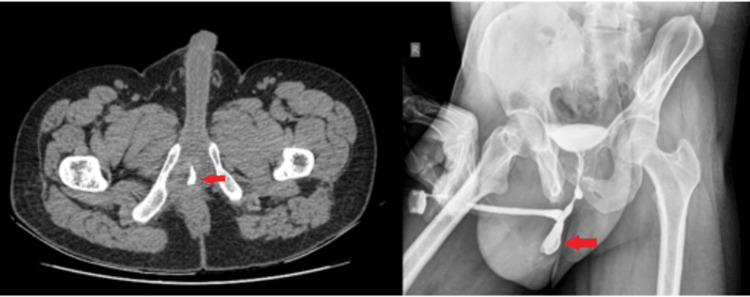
(Left) Computed tomography image suggestive of diverticulum with calculus. (Right) AUG image suggestive of diverticulum containing calculus AUG: ascending urethrogram
